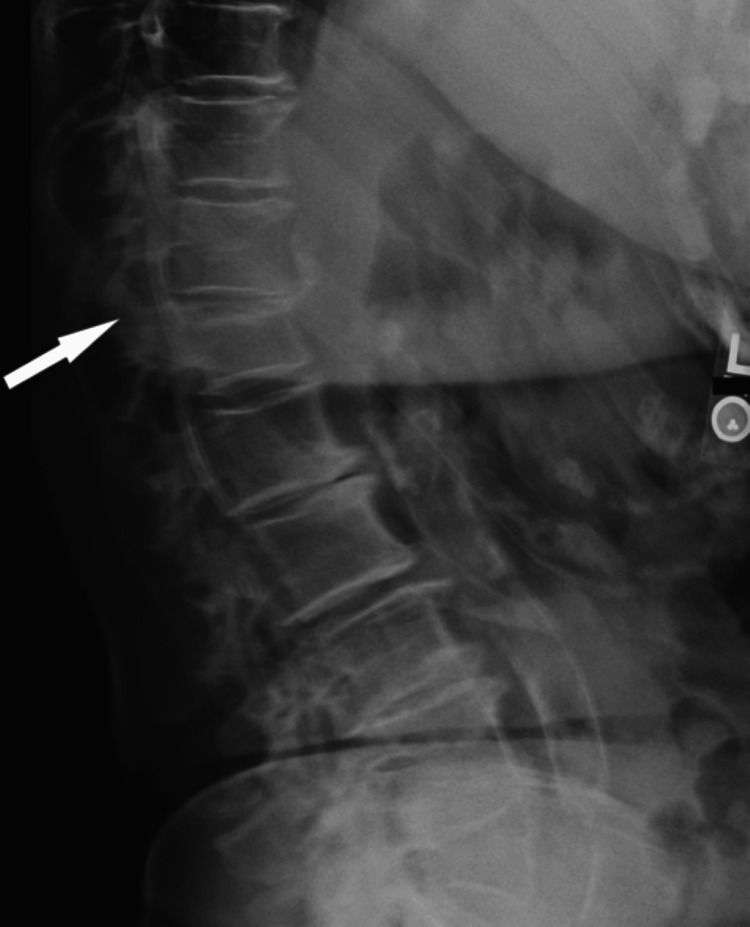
X-ray showing an acute upper endplate vertebral compression fracture at T12 (arrow)
